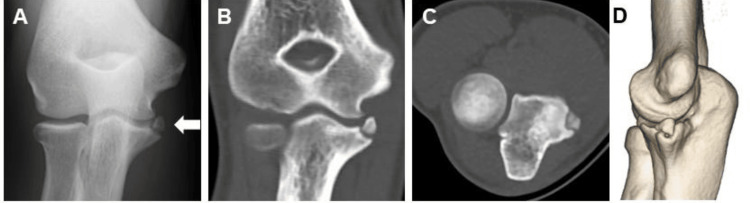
Preoperative radiographic and CT findings of case 1 A. Initial anteroposterior radiograph showing a small bony fragment at the ulnar sublime tubercle (white arrow). B. Coronal CT image. C. Axial CT image. D. Three-dimensional CT reconstruction.
